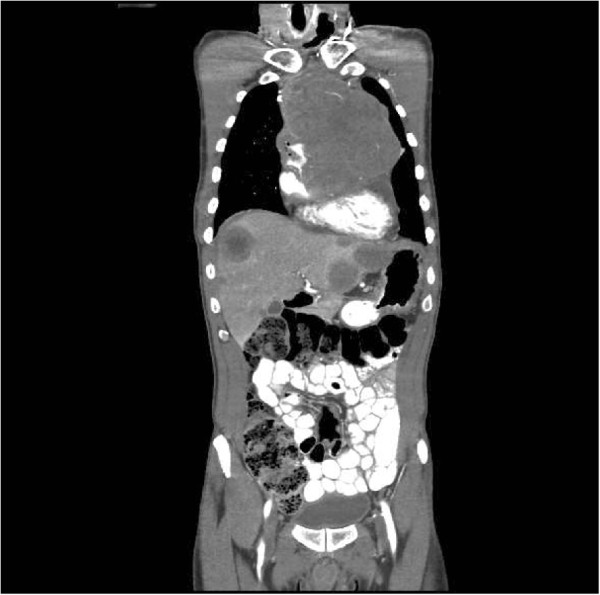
CT scan abdomen showing multiple enhancing hepatic lesions.
